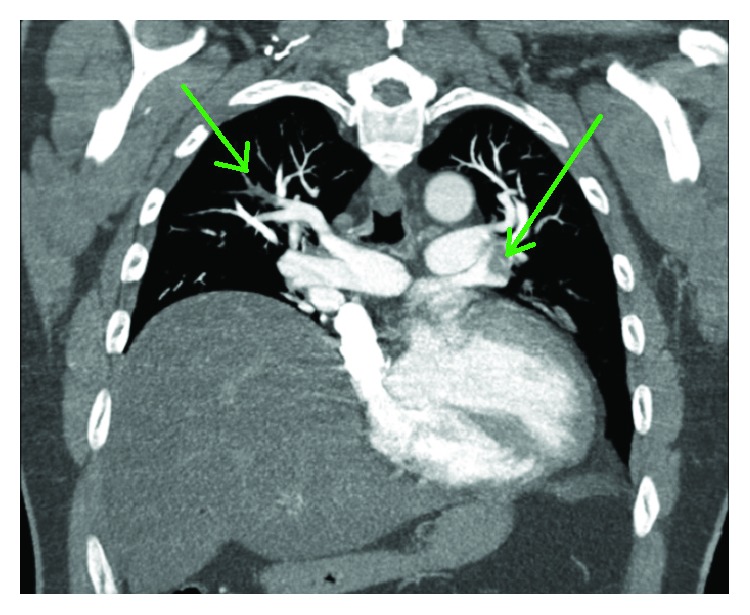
Computed tomography pulmonary angiogram revealed bilateral pulmonary emboli (green arrows).
